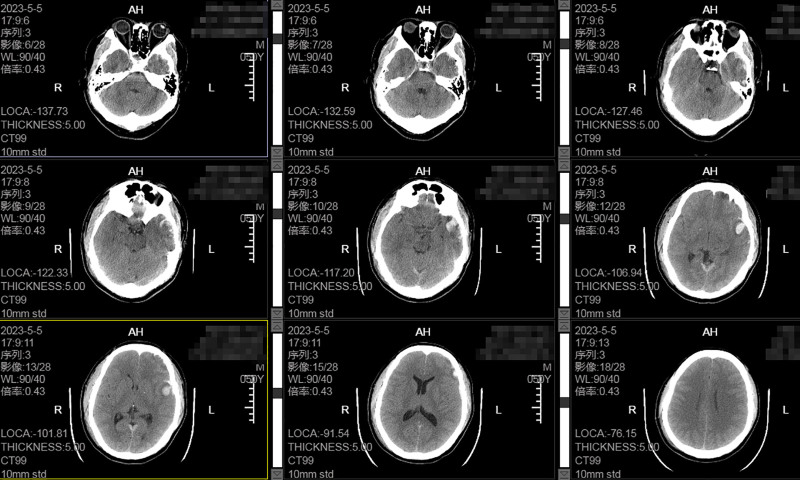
The initial CT scan upon admission revealed a traumatic subdural hematoma in the left frontotemporal region. The intracranial condition was stable, with no significant mass effect or midline shift, and there were no surgical indications. Based on these findings, conservative treatment was initiated. CT = computed tomography.
